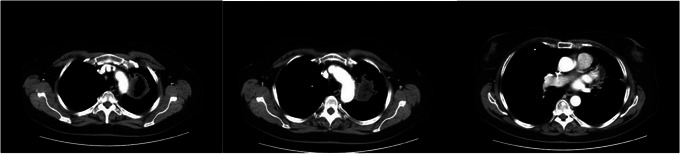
Sections of CT angiography demonstrating left mainstem bronchus involvement, pulmonary artery occlusion, and pericardial involvement of fungal mass.
